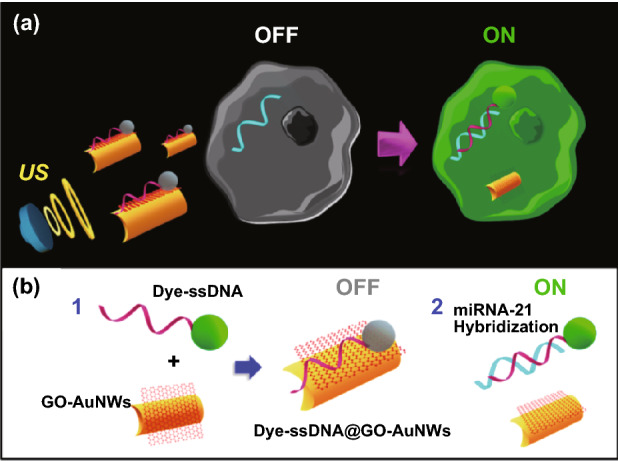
a Intracellular detection of miRNA in intact cancer cells using ultrasound (US)–propelled nanomotors. b the “OFF–ON” fluorescent switching system for the specific detection of miRNA-21 in intact cancer cells, and steps involved immobilization and quenching of the dye fluorescence. Adapted from Ref. [91] with permission, copyright American Chemical Society, 2015
